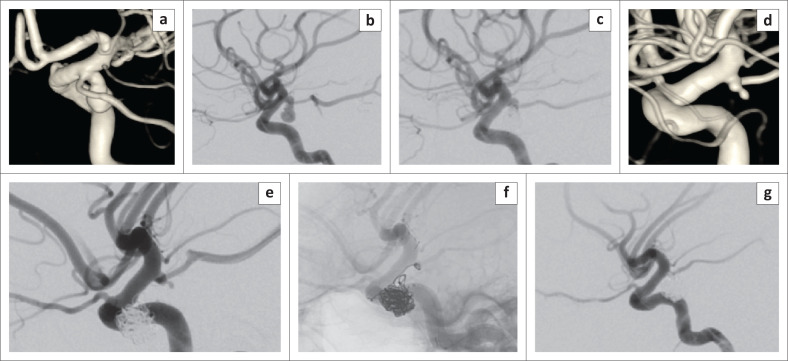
A 27-year-old woman presented with subarachnoid haemorrhage (SAH) and (right) third cranial nerve palsy. Computed tomography angiogram revealed bilateral common clinical presentation of posterior communicating artery aneurysms. The three-dimentional angiogram of the (right) internal carotid artery shows the larger symptomatic aneurysm (a). Lateral view before (b) and after (c) simple coiling. 3D angiogram of the (left) internal carotid artery shows the small, incidental mirror common clinical presentation of posterior communicating artery aneurysm with a relatively wide neck (d). This was treated 3 months later with a stent-assisted coiling (e and f). Control run (g) shows complete occlusion of the aneurysm with normal filling of the internal carotid artery and preservation of the common clinical presentation of the posterior communicating artery.
